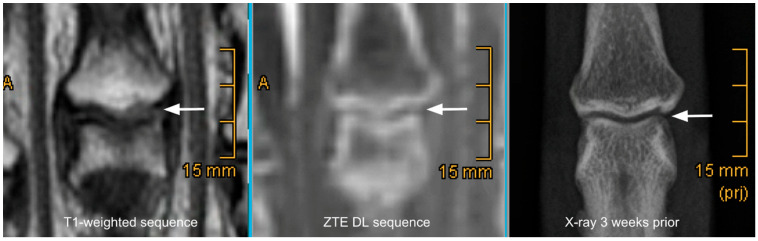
Forty-nine-year-old female with recurring arthralgias and edema in the hands, imaged with ZTE DL and T1-weighted MRI, with a corresponding radiograph obtained 3 weeks earlier. The joint space at the third proximal interphalangeal joint is better visualized using ZTE DL.
